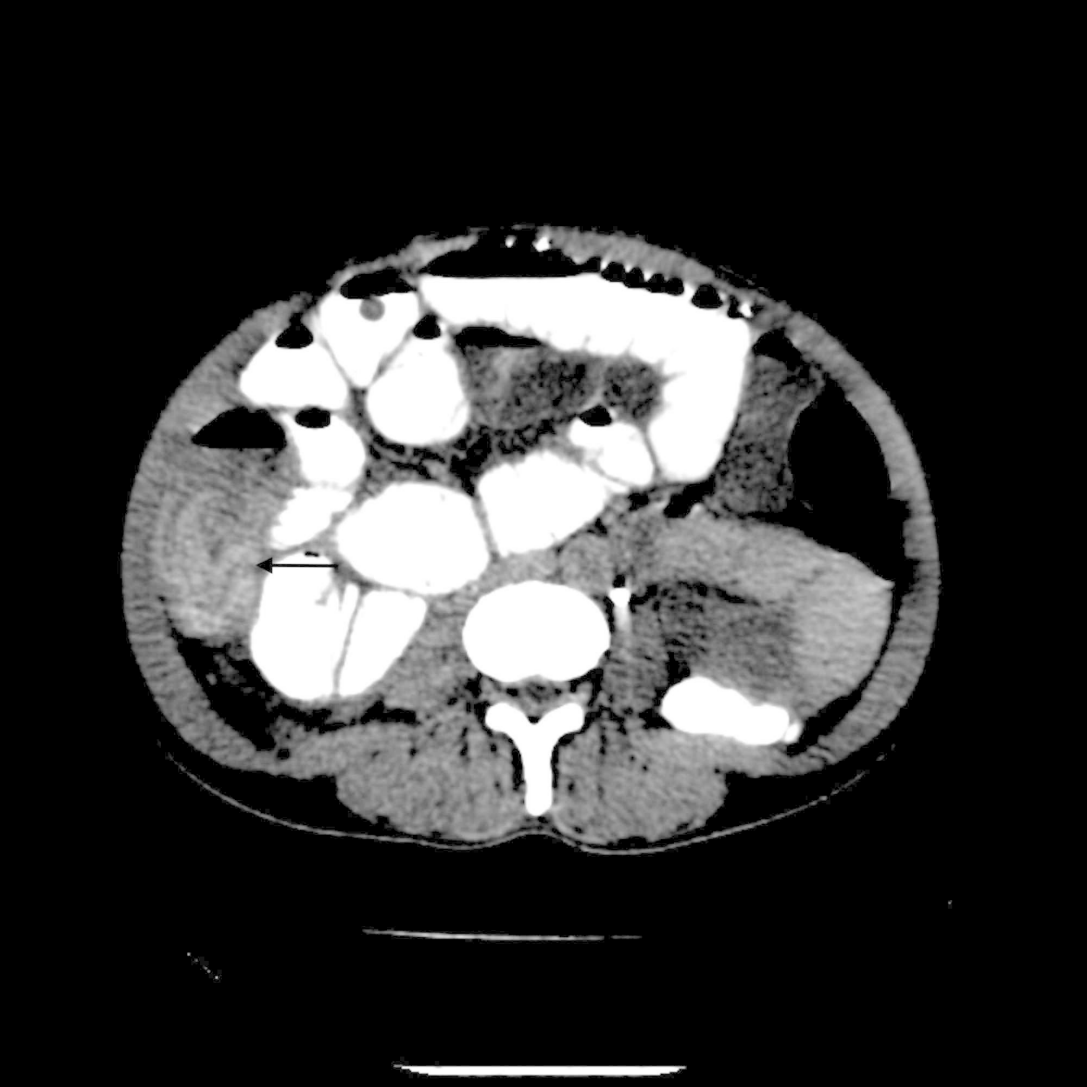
Axial CT image shows membranes within the ascending colon. Black arrow depicts membranes within the colon.
